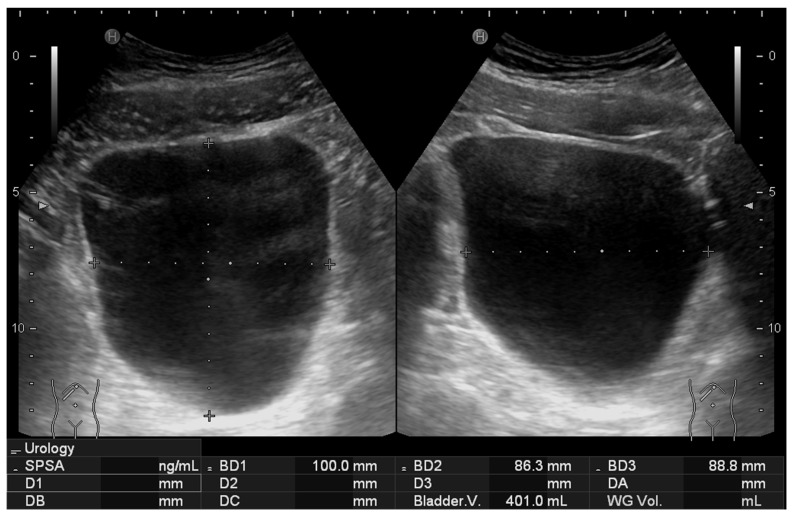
Representative 2D ultrasound image obtained using the standard method, showing the volume estimation at the bottom based on manual caliper measurements.
